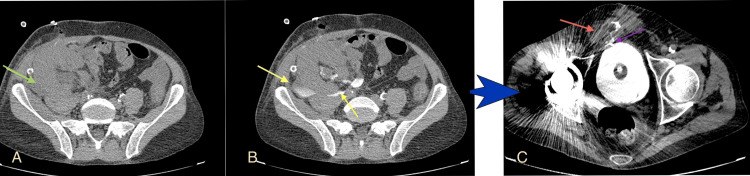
Leak at the level of the anastomosis of the renal pelvis and the native ureter (A) Axial multidetector computed tomography (MDCT) image before contrast administration reveals a low-density fluid collection around the transplant kidney (green arrow); (B) Axial MDCT image after bladder infusion with diluted solution shows a high-density collection outside the collecting system (yellow arrows) and around the transplant; (C) Axial image in the same patient after the Politano-Leadbetter ureteric reimplantation shows the distal part of the ureteric stent (purple arrow) and a remaining leak, this time from the ureter-bladder anastomosis (red arrow), with fistula formation with the lower abdominal wall. Image source: Department of Radiology, Ippokrateio General Hospital of Thessaloniki. Permission obtained for reproduction.
